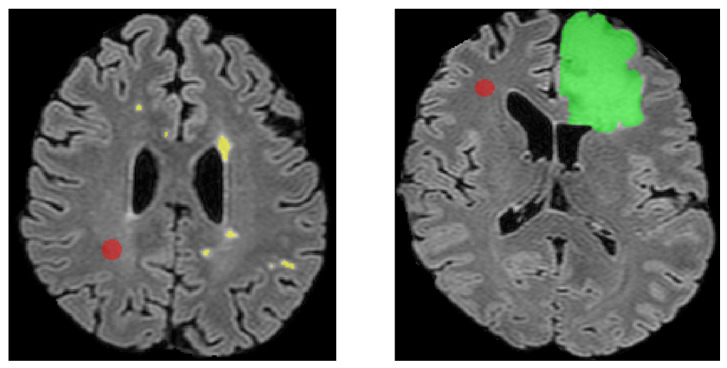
Two different patient examination slices from FLAIR modality from the validation dataset. (Left): Whiter matter hyperintensity GT is depicted with yellow color and the Healthy Reference VOI with red. (Right): Tumor GT is depicted with green and the Healthy Reference VOI with red.
